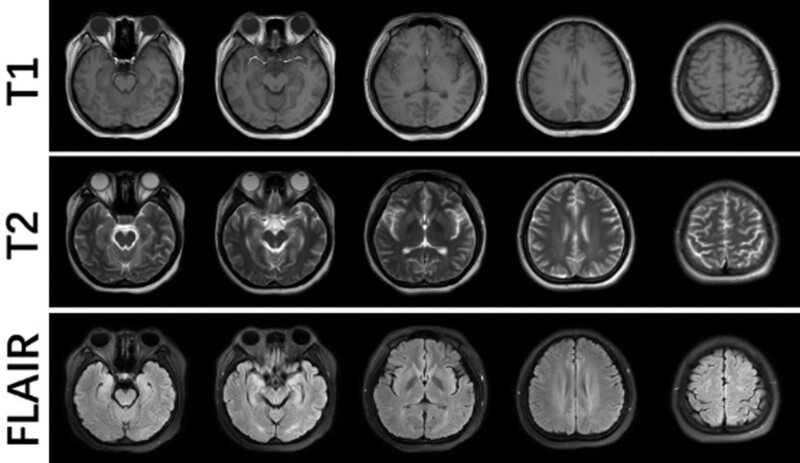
Eleven days after the first MRI scan, the second scan showed that the abnormal signal had completely disappeared. MRI = magnetic resonance imaging.
